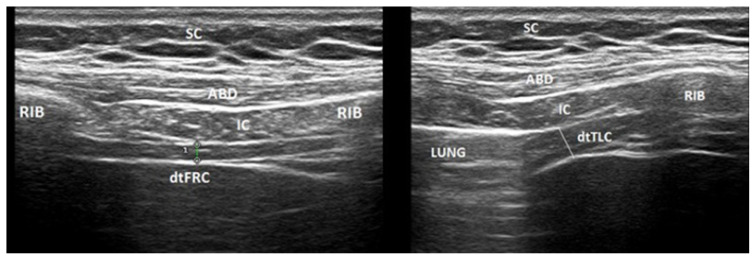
Ultrasonographic diaphragmatic thickness measurement at expiration (dt FRC) and maximal inspiration (dt TLC). ABD: Abdominal muscles, dt FRC: Diaphragmatic thickness at functional residual capacity, dt TLC: Diaphragmatic thickness at total lung capacity, IC: Intercostal muscles, SC: Subcutaneous tissue.
